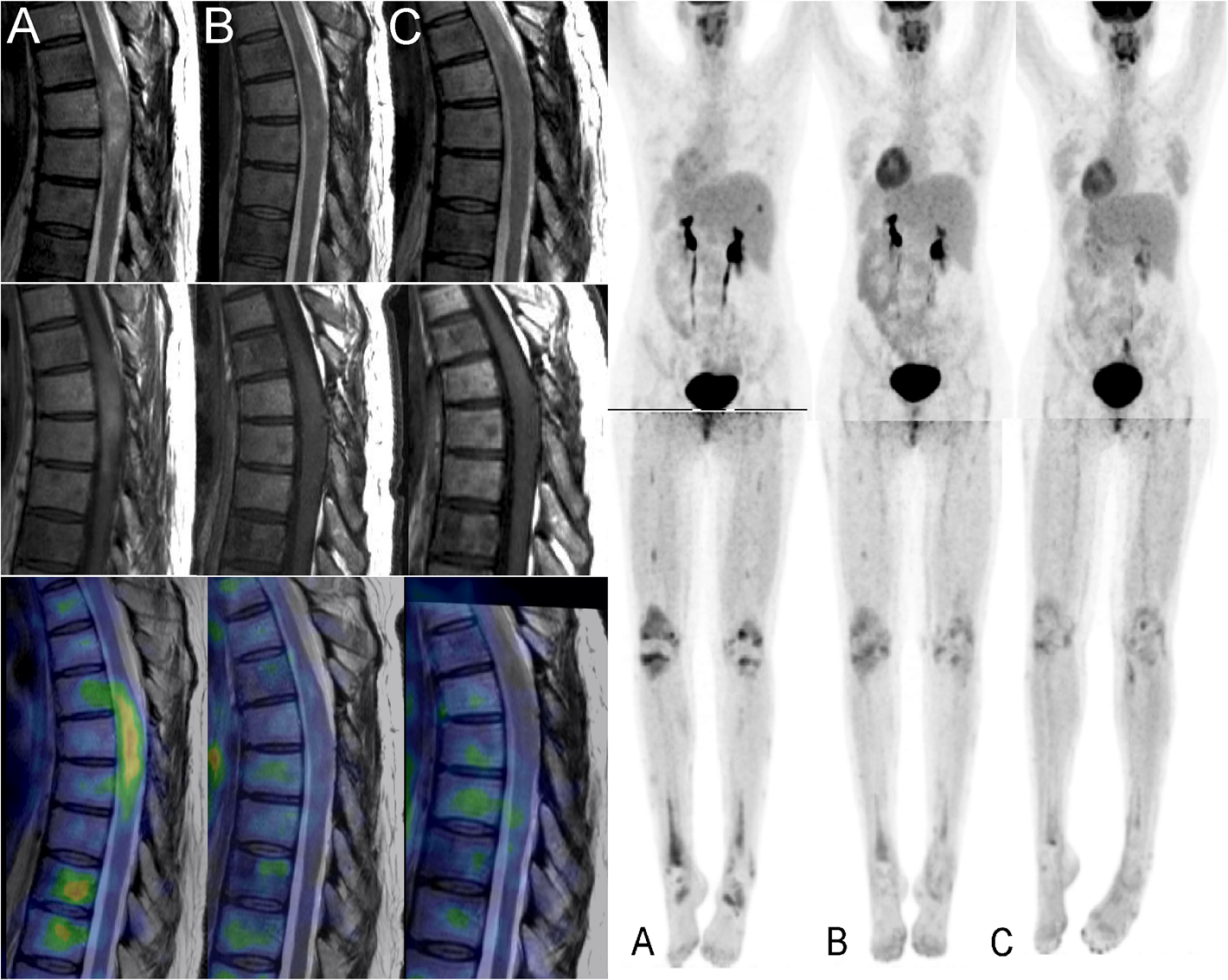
Treatment response. Left panel: Sagittal imaging of the spinal cord tumor at the level of the 2nd-7th thoracic vertebrae by T2-weighted MRI (upper row), T1-weighted, gadolinium enhanced MRI (middle row) and fusion with FDG-PET (lower row) taken at baseline (A), one month (B) and three months (C) of vemurafenib therapy. There is gradual regression of the intramedullary tumor’s size, edema (T2 signal) and contrast-enhancement. PET shows complete disappearance of FDG uptake in the tumor and substantial reduction in the bodies of the 6th and 7th thoracic vertebrae. Right panel: whole body FDG-PET taken at the same time points showing gradual regression of hot-spots in the patient’s skeleton and in particular the lower limbs.
